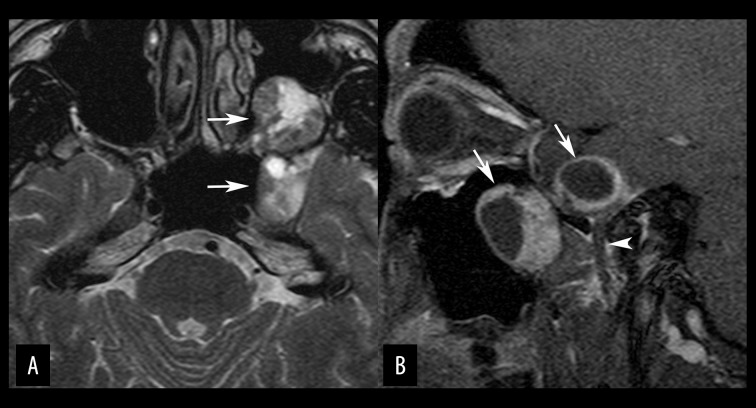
Trigeminal schwannoma in a 38-year-old man. (A) Axial, T2-weighted image show a dumbbell-shaped, partly cystic mass (arrows) extending from the left parasellar region to the left pterygopalatine fossa. (B) Sagittal, contrast-enhanced MR image with fat suppression shows both intra- and extracranial components of the tumor (arrows). The third branch of the trigeminal nerve seems normal (arrow head). Schwannoma of the second branch of the trigeminal nerve was confirmed on surgery.
